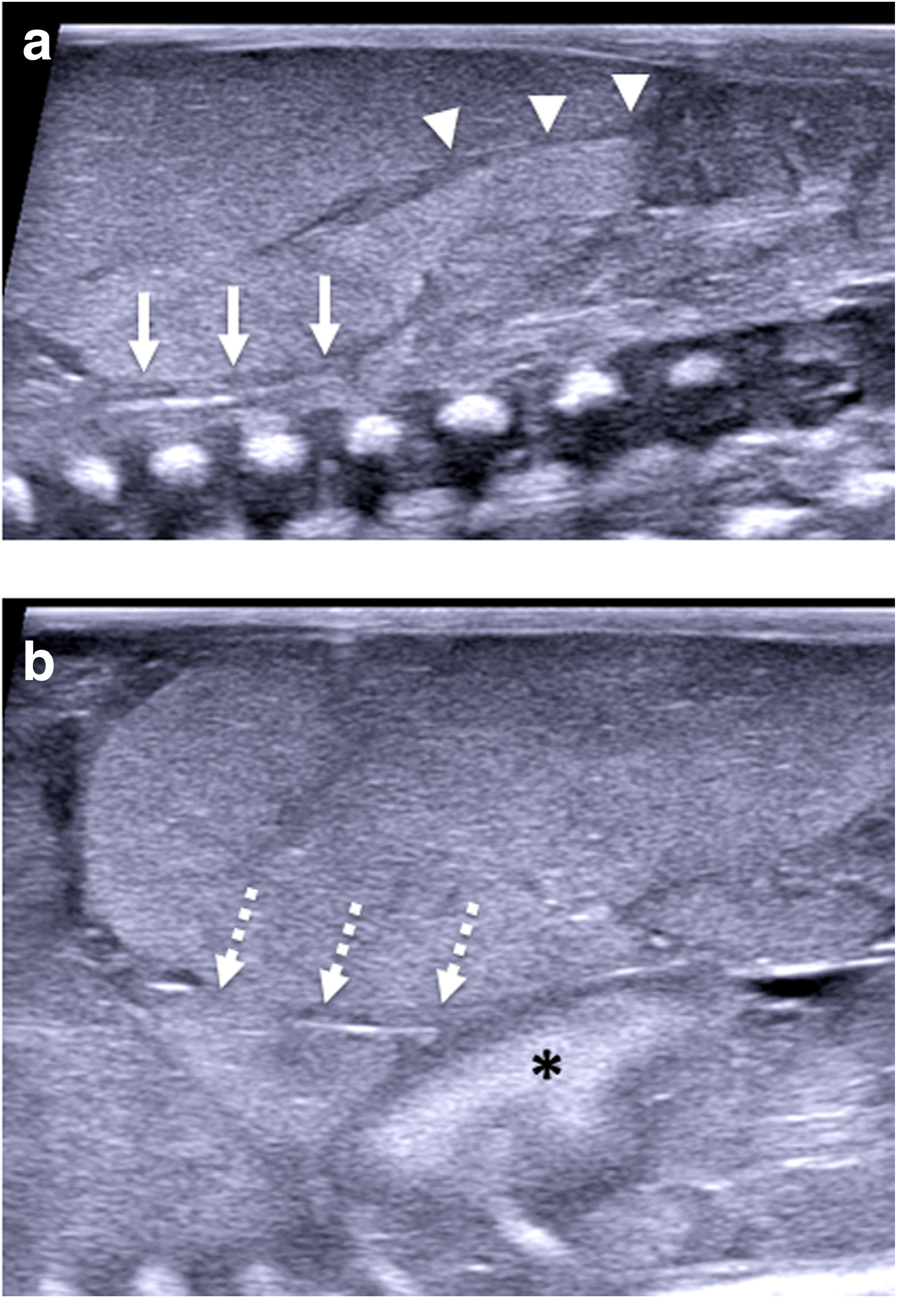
Sagittal postmortem ultrasound images of the upper abdomen in a 19-week gestational-aged foetus, obtained 8 days after death. Images through the abdominal aorta (solid arrows) (a) and ductus venosus (arrow heads) are both collapsed. The imaging through the inferior vena cava (dotted arrows) (b) show similar appearances. Another finding of note is the echogenic, prominent appearing right adrenal gland (asterisk). This is the usual expected appearance of the adrenal gland on perinatal postmortem ultrasound examinations
